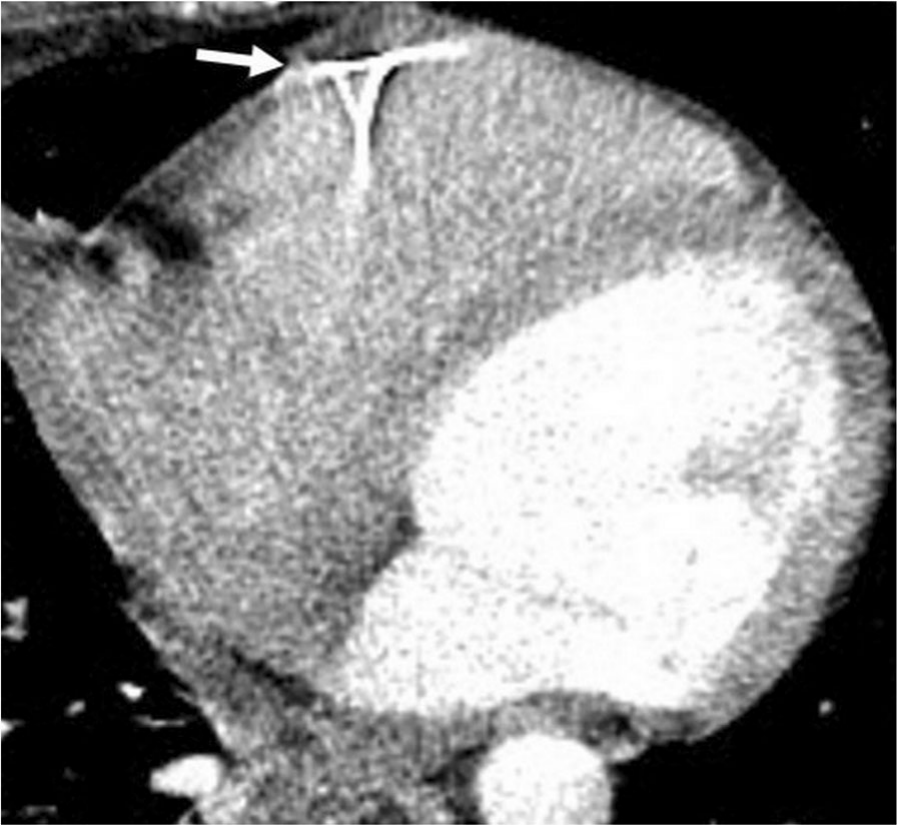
A 41-year-old war veteran. Axial contrast-enhanced CT image demonstrates a shrapnel fragment abutting the pericardium
